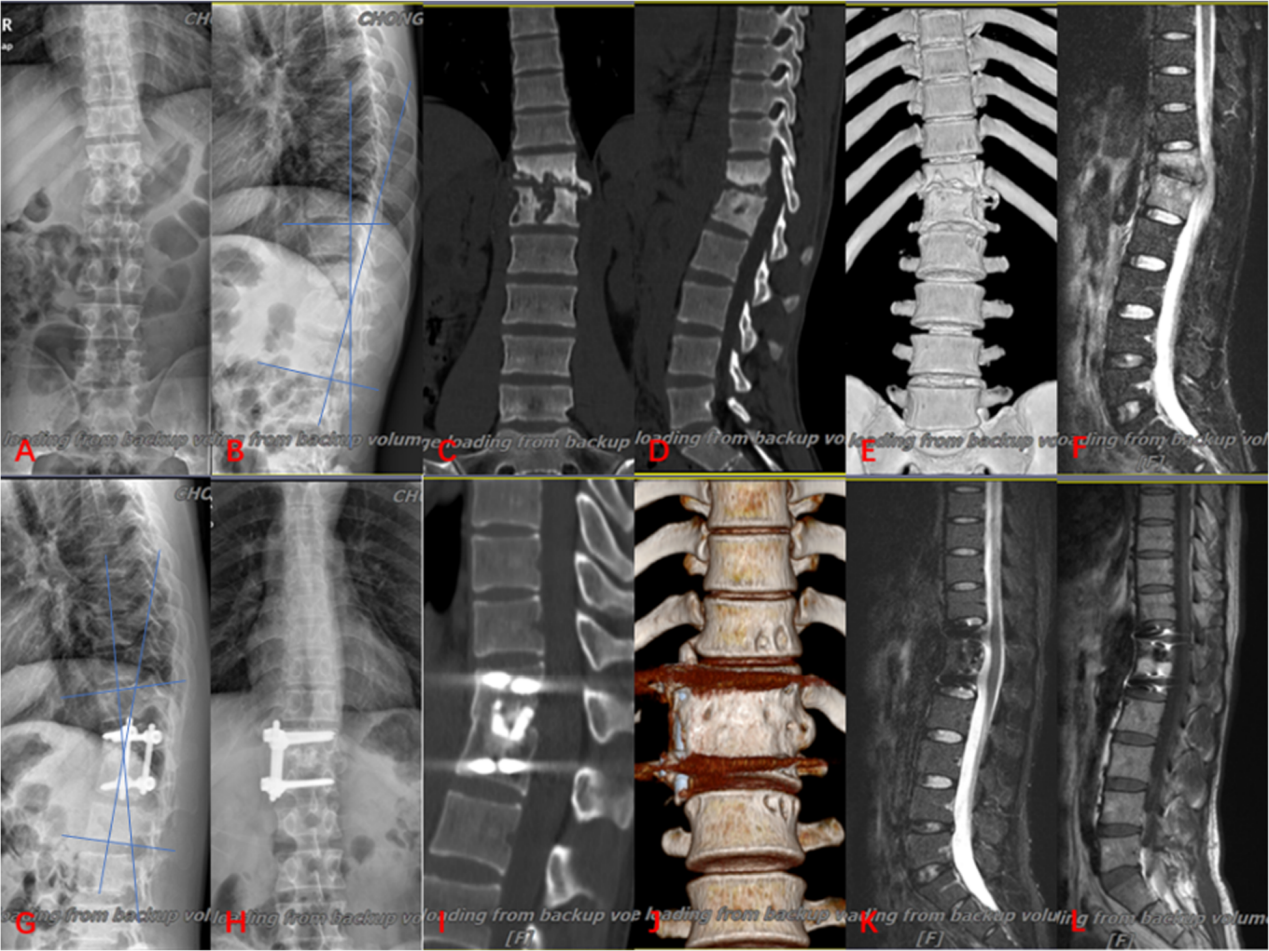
The graph showed a case underwent anterior debridement, bone grafting and screw-rods internal fixation. 25-year-old man with thoracolumbar junction (T12-L1) TB (a, b) preoperative anteroposterior and lateral X-rays; c, d preoperative computed tomography (CT); e preoperative 3D reconstruction of CT; f preoperative MRI; g, h) X-ray at 18-month postoperative; i CT at 24-month postoperative; j 3D reconstruction of CT at 24-month postoperative; k, l MRI at 18-month postoperative
